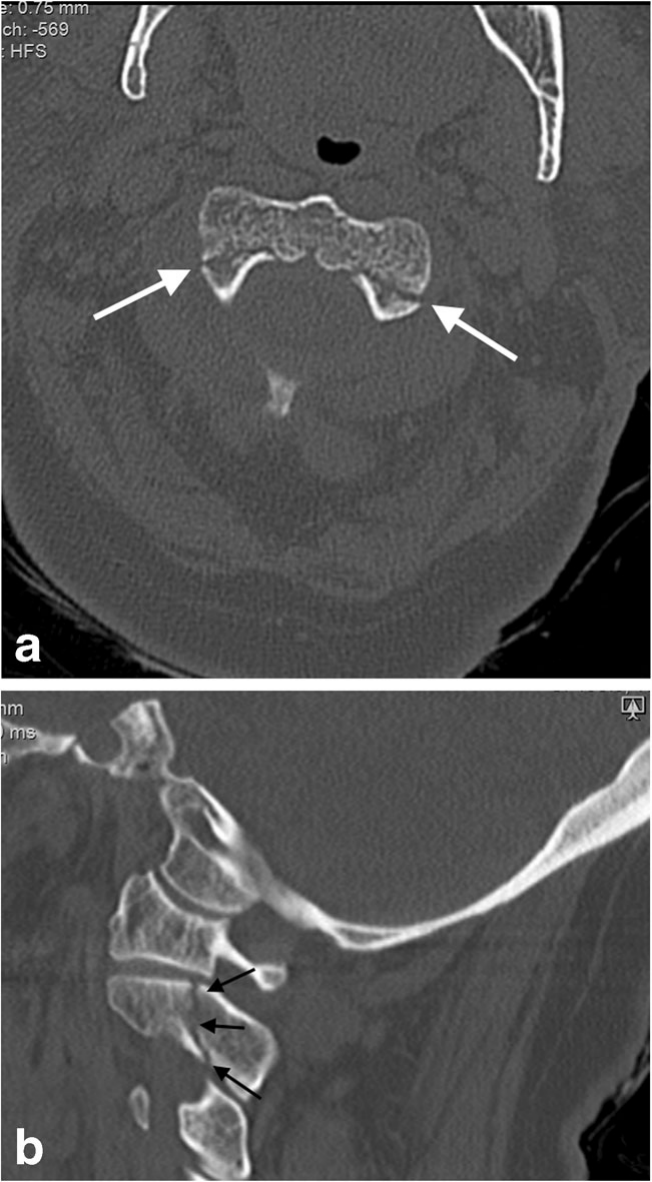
Classical hangman’s fracture in a 74-year-old male who fell down a flight of stairs: a axial CT image the C2 vertebra pars interarticulares demonstrating the typical fracture line locations in this type of classical hangman’s fracture (*arrows*); b sagittal reformatted CT image through the right pars interarticularis demonstrates the typical fracture line location (*arrows*) which was also evident in the left pars interarticularis (not shown)
